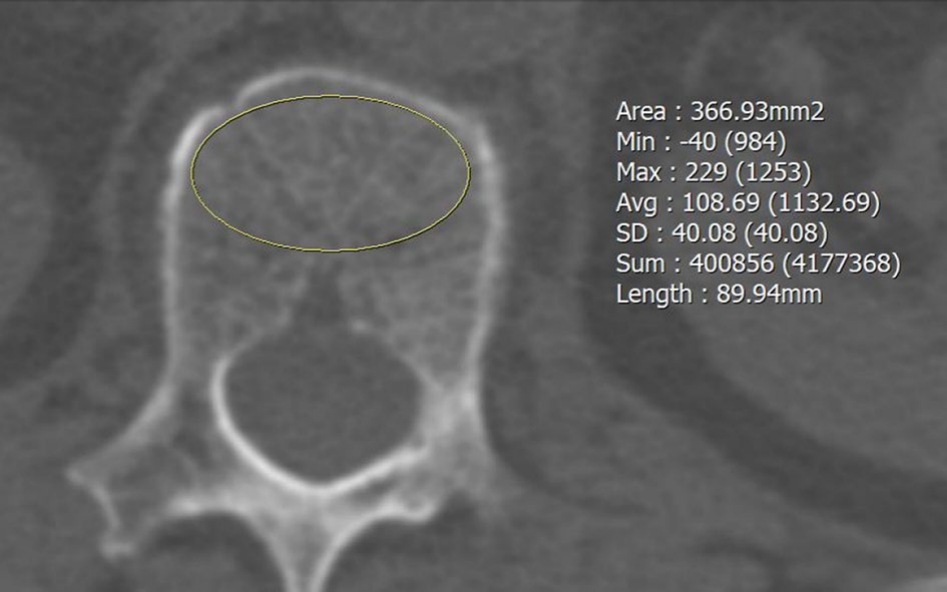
Example of CT Hounsfield unit (HU) measurement in an axial image of an upper instrumented vertebra at the mid-body level. The mean HU value was automatically derived by the picture archiving and communication system. *Min* minimum, *max* maximum, *avg* average, *SD* standard deviation.
